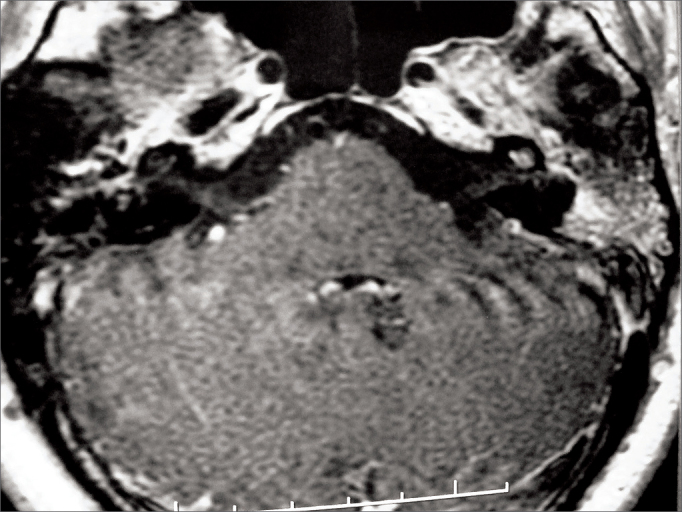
T1-weighed head MRI scan, axial view after contrast has been injected at the level of the inner ear canal; intense contrast uptake seen in the left ear's cochlea and mastoid cells.
